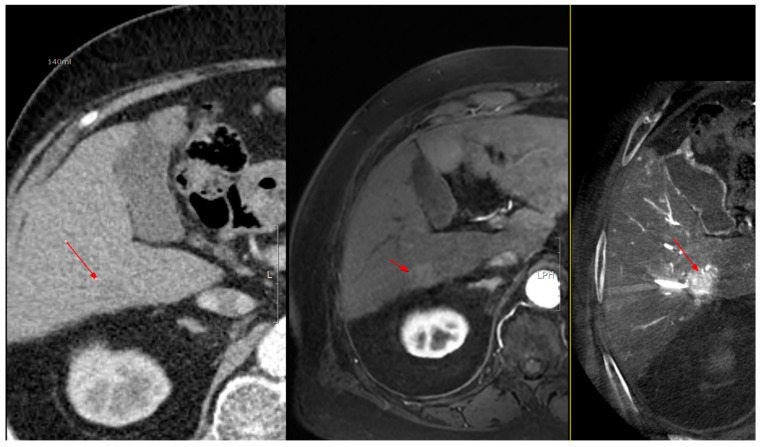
Images from another patient with hepatocellular carcinoma undergoing angio CBCT-guided RFA. All red arrows indicate the HCC mass. (Left): pre-procedural CT (portal phase). (Middle): pre-procedural MRI (arterial phase). (Right): intraprocedural angio CBCT. (Intra lesion − Intra background = 432.7 HU).
